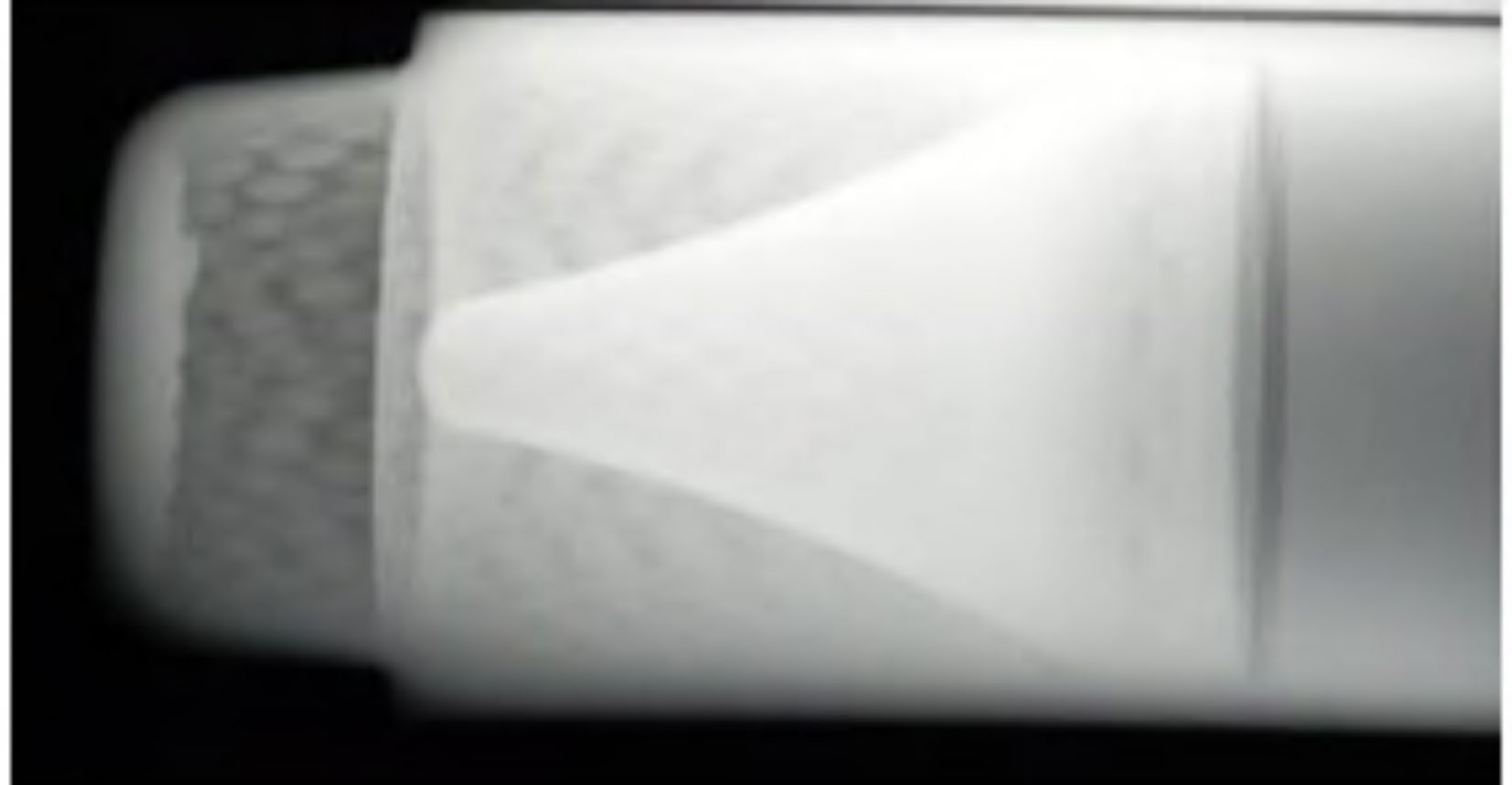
X-ray photograph of the explosive charge.
